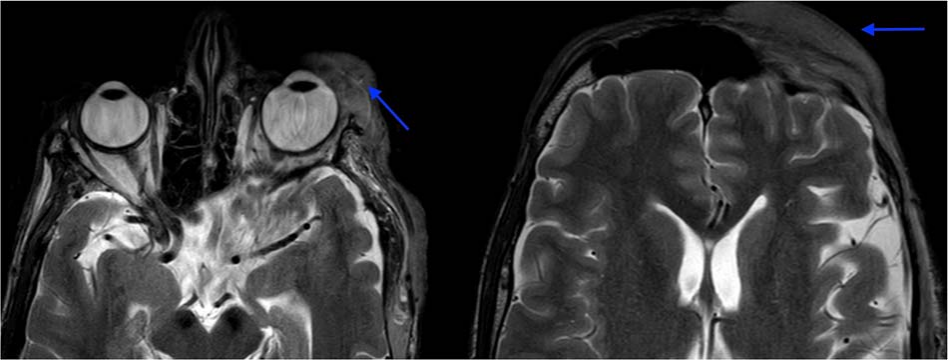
MRI orbit revealed enhancing skin thickening over the left frontal region, maxillary region, temporal region, supraorbital region, and over the left eyelids covering the eye.
